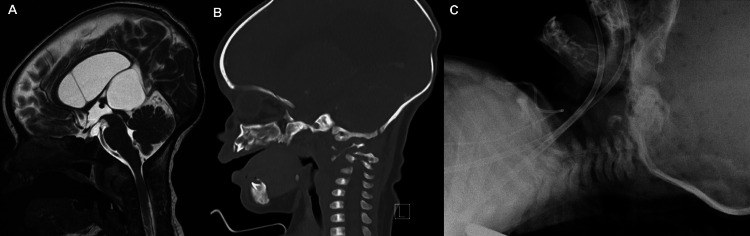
At first presentation. (A) T2-weighted brain image shows abscess formation involving the C1-C2 level with prevertebral enhanced soft tissue inflammatory changes. Significant bone destruction on the right side of C1-C3 and narrowing of the craniocervical junction are also evident. (B) Head CT shows the phlegmonous reaction in the retropharyngeal space with definite residual abscess collection as well as widening of predental space with atlantoaxial subluxation. (C) Cervical X-ray demonstrates widened atlanto-odontoid interval, basilar invagination of the upper cervical spine, and pre-atlantoaxial retropharyngeal soft tissue thickening.
